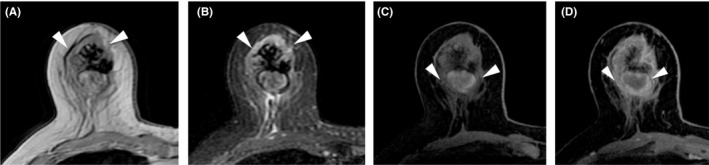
Magnetic resonance imaging. A, T1‐weighted images. The edge of mass is isointense (arrowhead). B, T2‐weighted images. The edge of mass is hyperintense (arrowhead). C, Contrast‐enhanced T1‐weighted images in the early phase (arrowhead). D, Contrast‐enhanced T1‐weighted images in the late phase (arrowhead)
